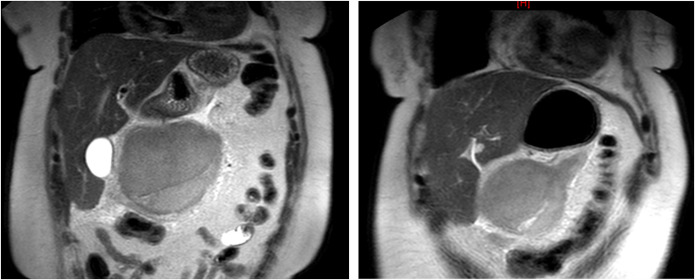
Magnetic resonance imaging of the abdomen with and without contrast: 13 × 10-cm circumscribed, hypovascular mass located inferior to the stomach with adjacent fat stranding, possibly arising from the greater curvature of the stomach. Distinct from the pancreas and colon. Likely differential and considerations include gastrointestinal stromal tumor, among other etiologies such as leiomyoma.
